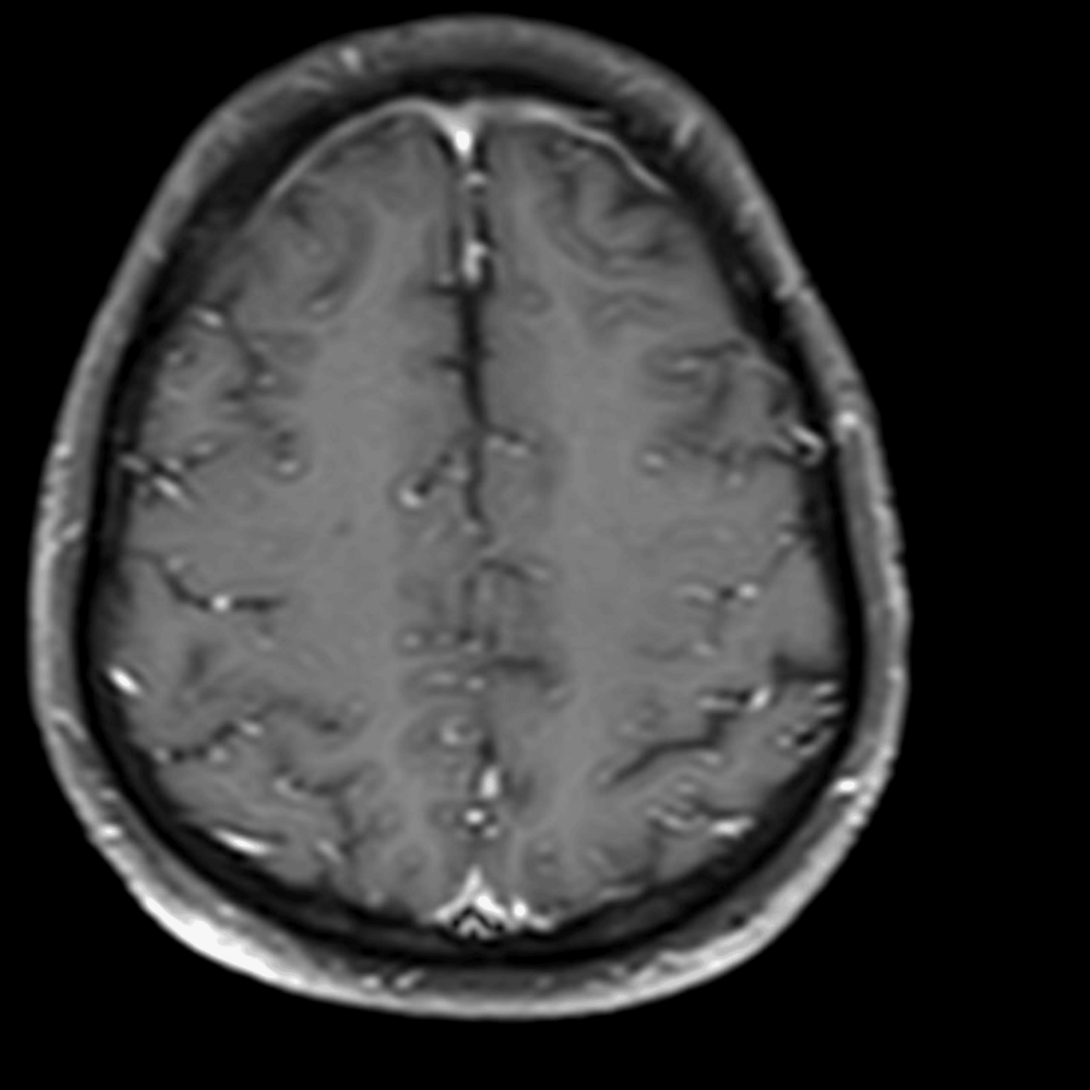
Post-contrast T1-weighted axial image demonstrates subtle leptomeningeal enhancement, particularly in the cortical sulci of the frontal lobes, suggestive of meningeal inflammation
